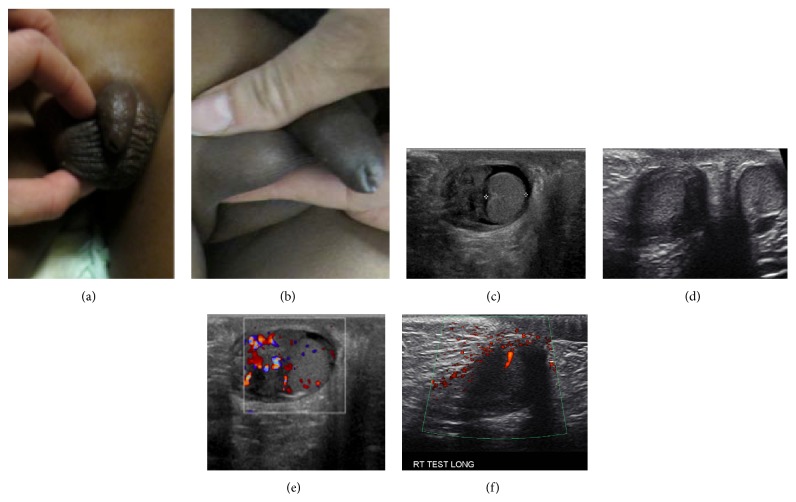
Comparison of right testes before and after treatment with exam and images. (a) Enlarged right testis before treatment, (b) normal sized right testis after treatment. Both testes (not shown) on follow-up exam were equal in size and volume. (c) Right testicular US before treatment. (d) Right testicular US after treatment: follow-up scrotal ultrasound demonstrated normal appearing right testis and epididymis with equal volume and size compared to left. (e) Right testicular Doppler before treatment shows hyperemia. (f) Right testicular Doppler after treatment shows normal Doppler flow.
